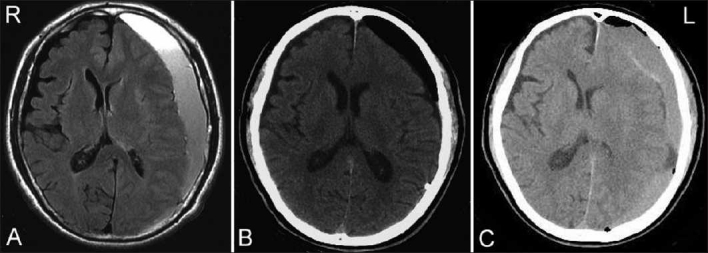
MRI and CT images of case 3. (A) MRI on admission showing left CSH. (B) CT scan after irrigation and drainage. (C) CT scan 13 days after the surgery, showing recurrence of left CSH
